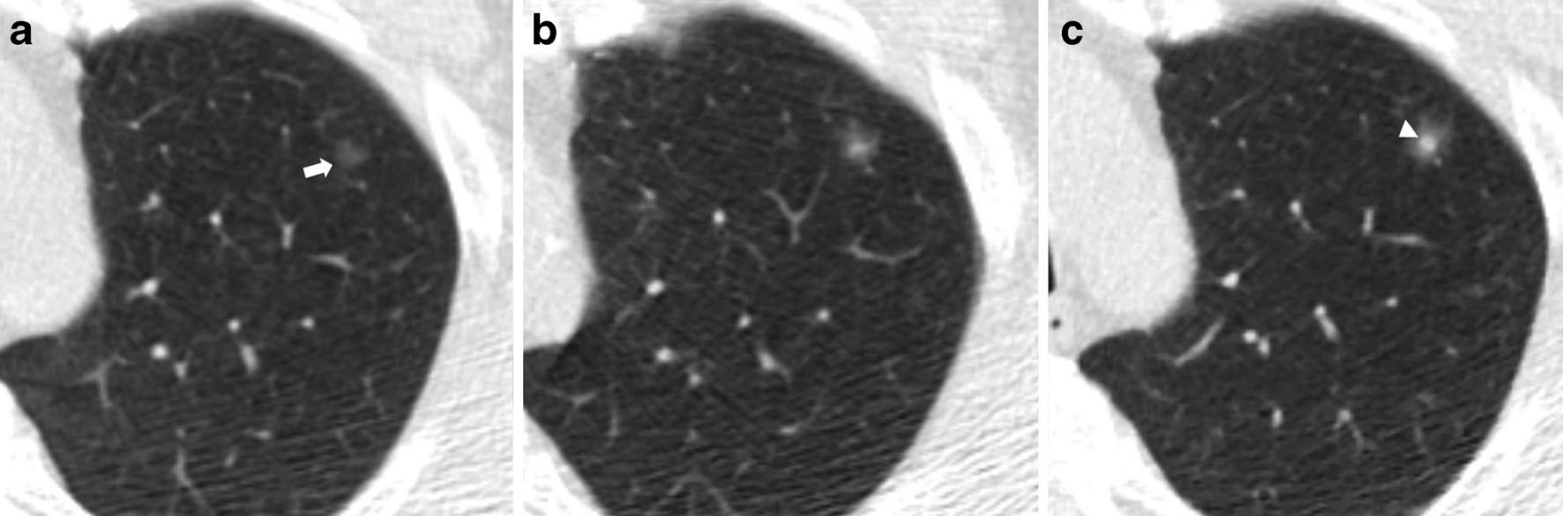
Increased attenuation of a small lung adenocarcinoma over 3 years in a 62-year-old man. (a) Low-dose CT image show a 5-mm non-solid nodule of the upper left lobe (arrow). (b) Follow-up CT obtained 1 year later show a minimum increment of axial diameter and a slight increase of nodule attenuation. (c) Two years later the progression to a part-solid nodule is obvious. The development of a central solid component (*arrowhead*) within the nodule can be observed. An increase in pulmonary nodule attenuation at LCDT follow-up, the development or increment of a solid component within the pulmonary nodule should raise suspicions of malignancy, even if the nodule size is stable. Typically, this appearance is suggestive of a peripheral lung adenocarcinoma
